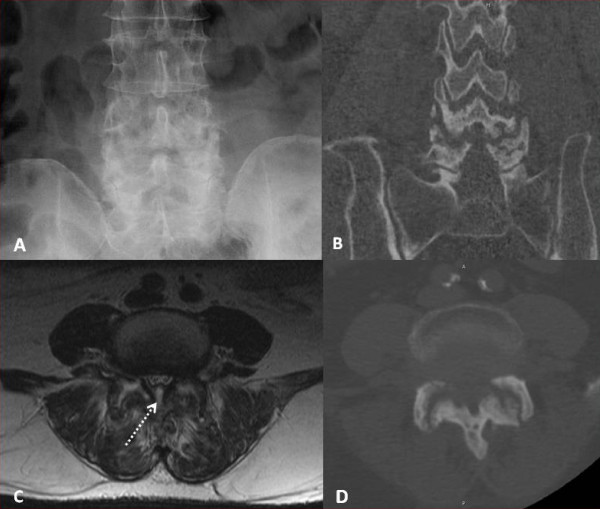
Advanced degenerative changes of the L4/5 facets are seen on these AP radiograph and CT scan images. (1A, B and D) Note the subchondral sclerosis and cystic changes. The axial T2 MRI image shows a focal fluid-like collection in bilateral L4/5 facet joints with contiguous extension into the midline dorsal epidural space (dotted line) (1C). Additionally, there is heterogeneous increased signal in the paravertebral muscles. There is no evidence of spondylodiscitis or paravertebral muscle abcess.
